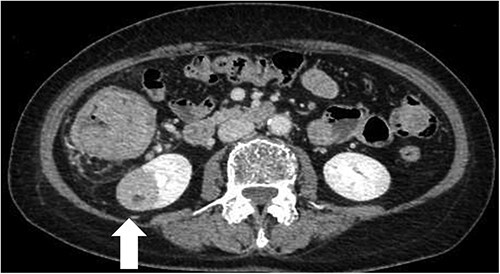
CT scan of the renal tumor in the lower pole of the right kidney (arrow).
